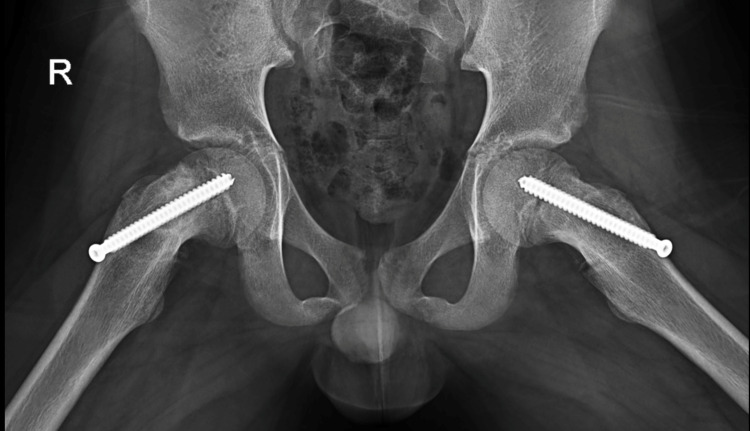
Nine-month follow-up frog bilateral hip X-rays.
